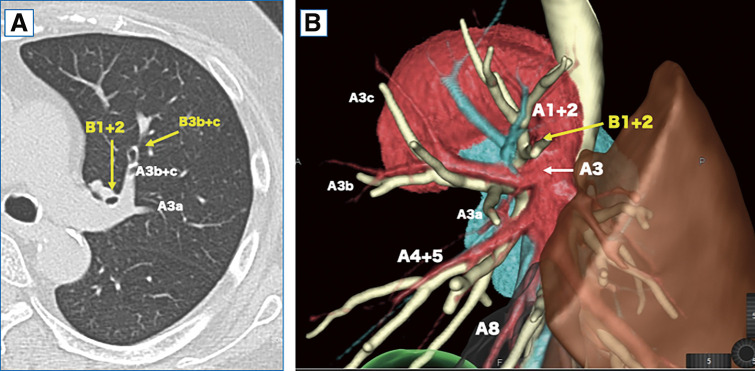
Thin-slice computed tomography (CT) and Three-dimensional CT findings. (A) Thin-slice computed tomography (CT) findings: The left A3 exhibited vascular branching, with all branches originating from the interlobar pulmonary artery. (B) Three-dimensional CT findings: The left A3a branched from the A3 stem, while A3b and A3c branched at the periphery. The lingular artery branched independently from the interlobar pulmonary artery.
